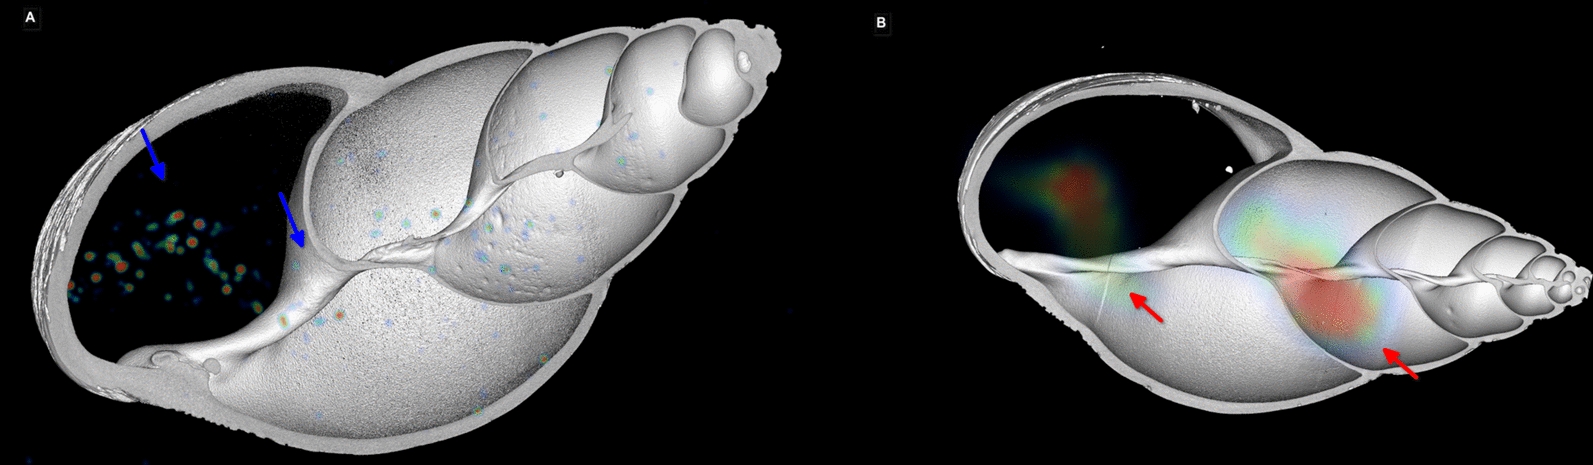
PET/CT image of two *Lissachatina fulica* snails scanned 10 min after orally feeding ^18^F-FDG-radiolabeled *Crenosoma striatum* larvae. A, Snail, top-view uptake in digestive tract up to the stomach, blue arrows. B, snail 2, top-view uptake in the digestive tract, red arrows
